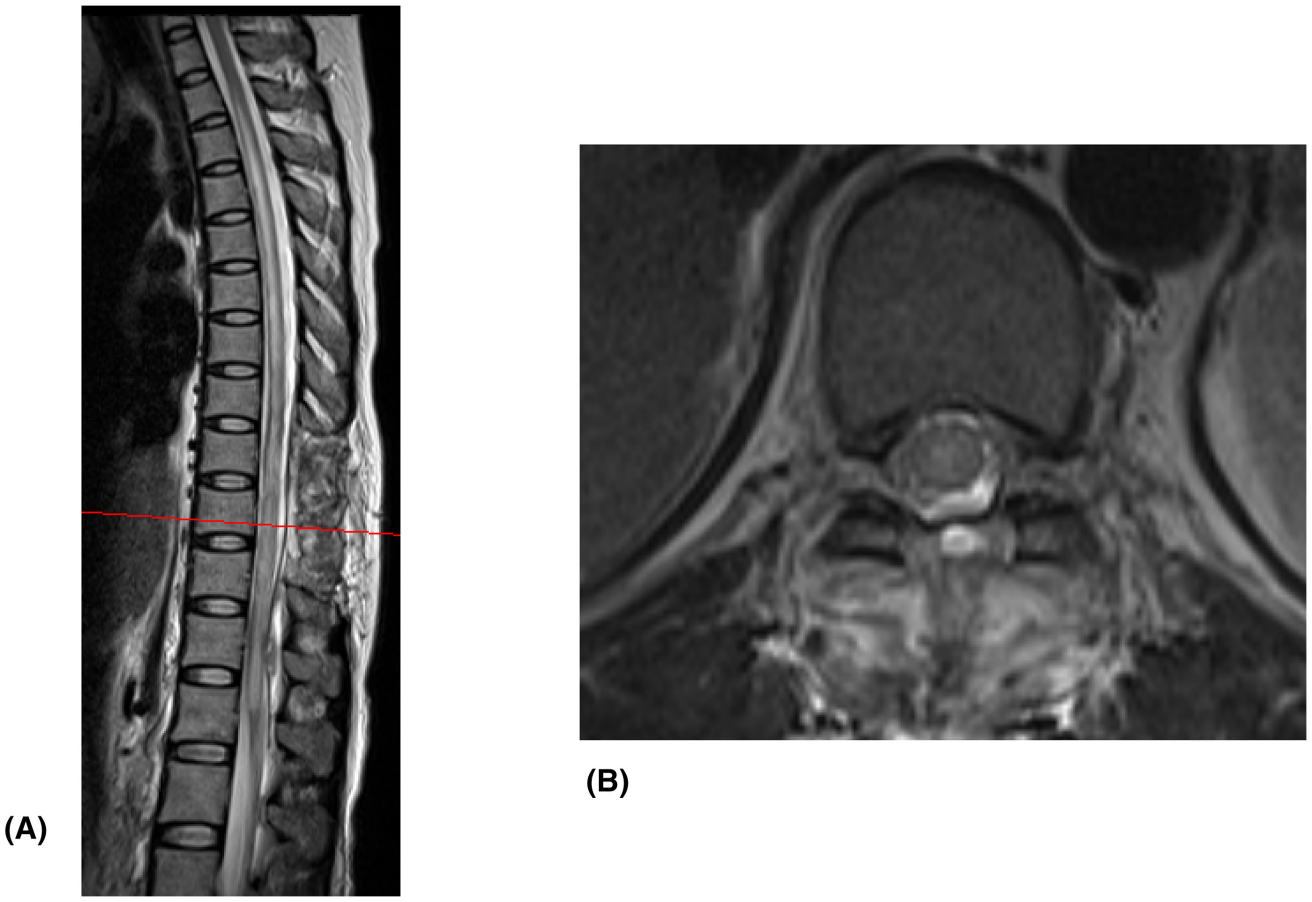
Magnetic resonance imaging (MRI) 3 days after surgery. (A) Sagittal and axial. (B) T2‐weighted MRI showing postoperative findings after laminectomy and hematoma removal, and decompression of the thoracic spinal cord. Extensive spinal cord edema (T2 to the conus medullaris) remains.
